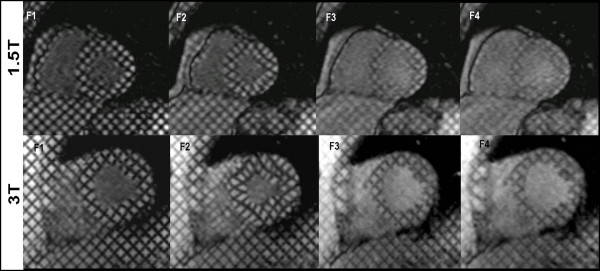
1.5 and 3T mid ventricular short axis tagging covering the cardiac cycle (F1-F4). Note better tag-tissue contrast and longer tag persistence (F4) on 3T MRI system.
